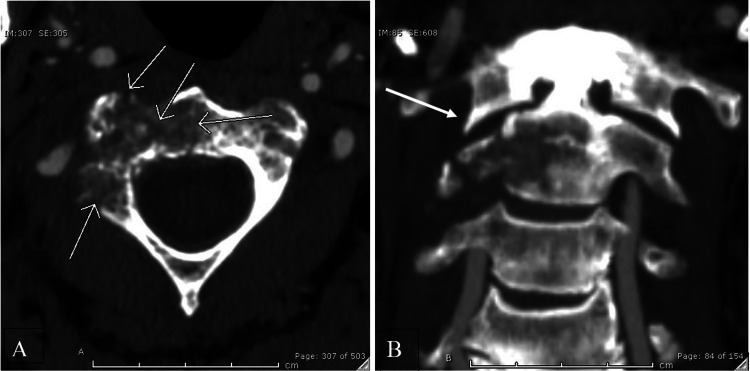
Axial (A) and coronal (B) contrast-enhanced computed tomography (CT) angiogram of the neck depicting the pathologic vertebral body lesion Figure A demonstrates lucency and cortical irregularity (arrows) in the right anterior aspect of the C2 vertebral body. There is asymmetric widening of the right atlanto-axial joint with cortical irregularity at C2 (thick arrow, B). This, along with lucency of the C2 right lateral mass, indicates pathologic fracture.
